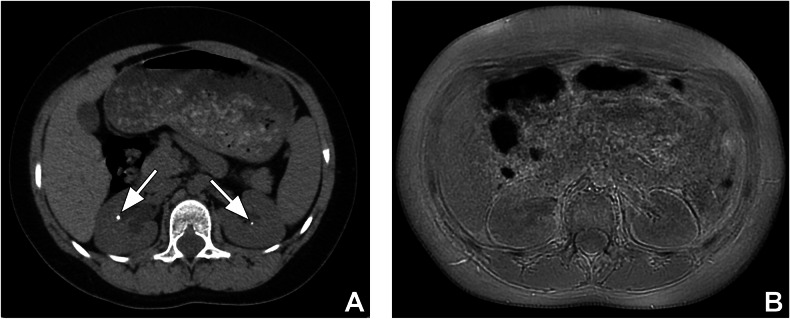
A 9-year-old boy with bilateral renal stones. A Axial non-enhanced CT image shows bilateral < 5 mm renal stones (arrows). B Failure to detect stones on axial ZTE-MR image
